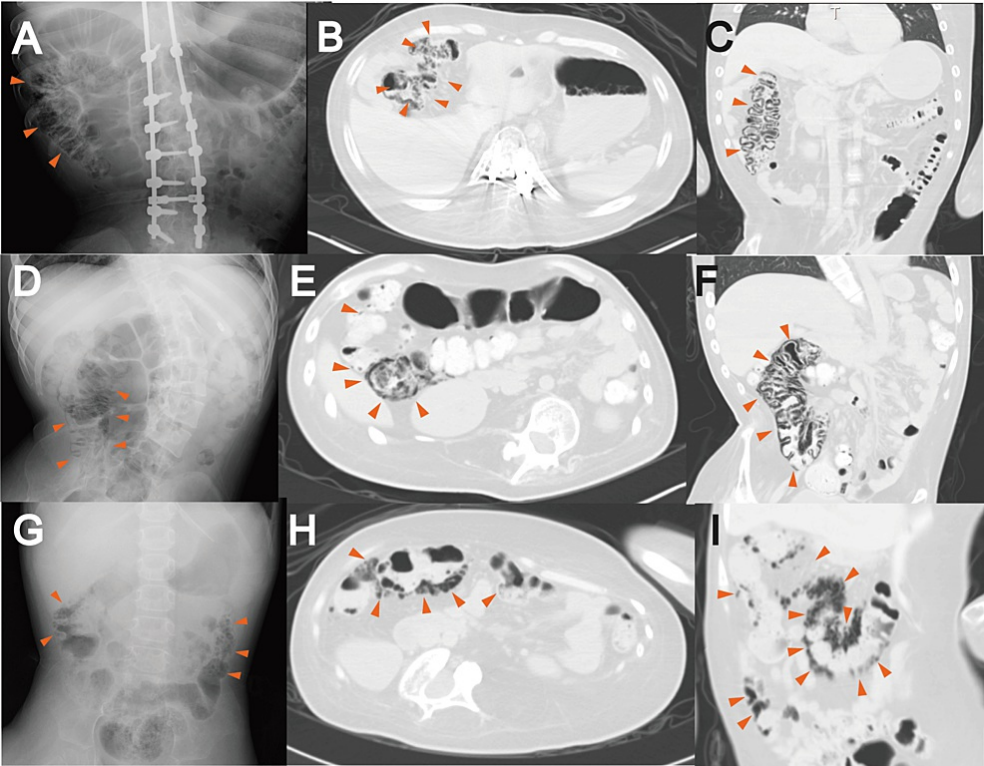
Abdominal radiographs and computed tomography (CT) images of the cases (A–C) Case 1, (D–F) case 2, (G) case 3, and (H–I) case 4. (A), (D), and (G) Abdominal X-ray. (B), (E), and (H) Axial CT scan images of lung window. (C), (F), and (I) Coronal CT scan images of lung window. Linear gas shadows were observed (red arrowheads).
